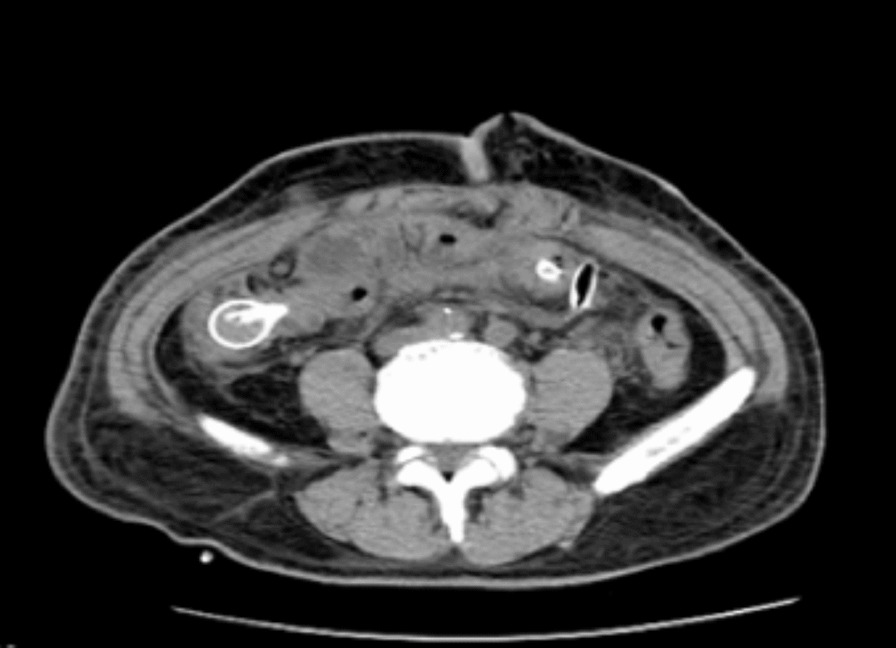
Abdomen computed tomography (CT) scan after surgery. The prior long intestinal tube was sent to the cecum and also used to release the entrapped residual small intestine
